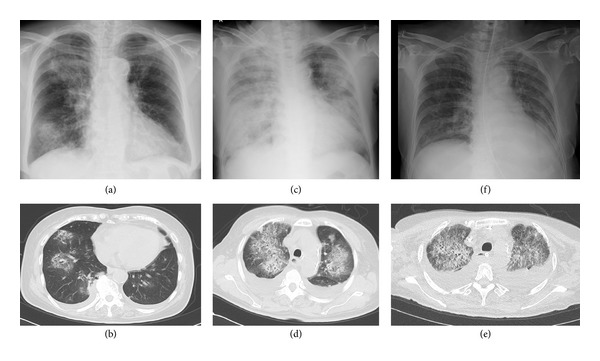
(a), (b) Chest X-ray and CT on admission showed bilateral central opacities, which are more confluent in the right upper and lower lung zone than elsewhere. There is slight cardiomegaly. (c) The radiograph obtained after intubation on the 10th day showed increased confluence of the opacities. The right hemidiaphragm is partially obscured. (d) Chest CT scan on the 10th day showed bilateral ground-glass opacities corresponding to the central and perihilar opacities and both small pleural effusions. (e) Chest CT scan on the 36th day showed progressed bilateral ground-glass opacities, confluent, and consolidated opacities, and a large quantity of pleural effusions in both lung fields. (f) Chest X-ray on the 92nd day showed the disappearance of infiltrative shadows in both lung fields.
